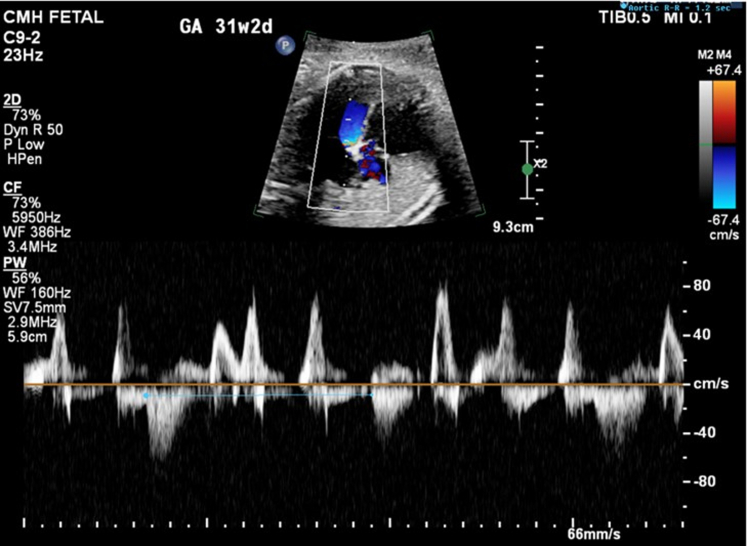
Doppler Interrogation of the Left Ventricular Mitral Valve Inflow and LVOT Flow The blue cursor is placed at the onset of aortic flow and measures an aortic R-R interval of 1.2 seconds, correlating to a fetal ventricular rate of 50 beats/min. The Doppler above the baseline is representative of the mitral valve inflow. There are both biphasic inflow Doppler patterns as well as high-velocity “a” waveforms seen corresponding to atrial contraction under conditions of abnormal ventricular filling properties. LVOT = left ventricular outflow tract.
